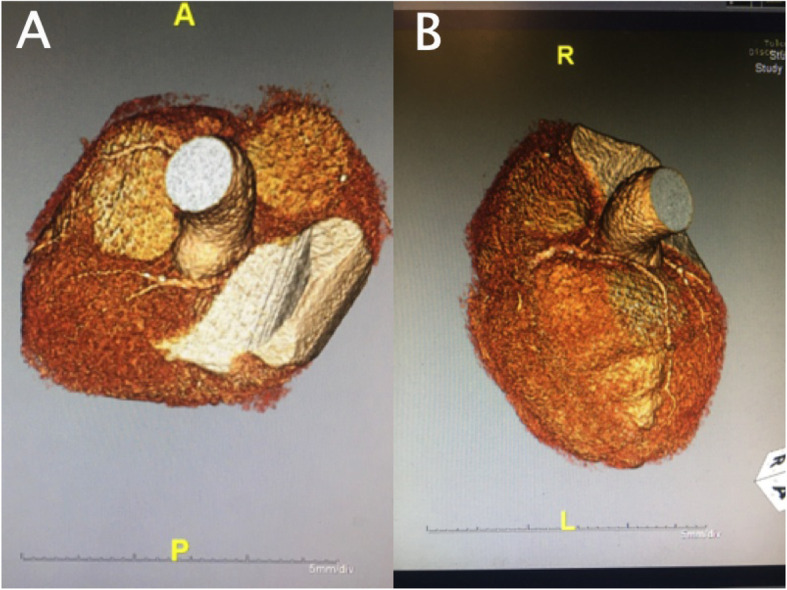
Two views (a and b) from CCTA imaging revealing the left anterior descending artery originating posteriorly and coursing between the aorta and left atrium. *CCTA* coronary computed tomographic angiography
